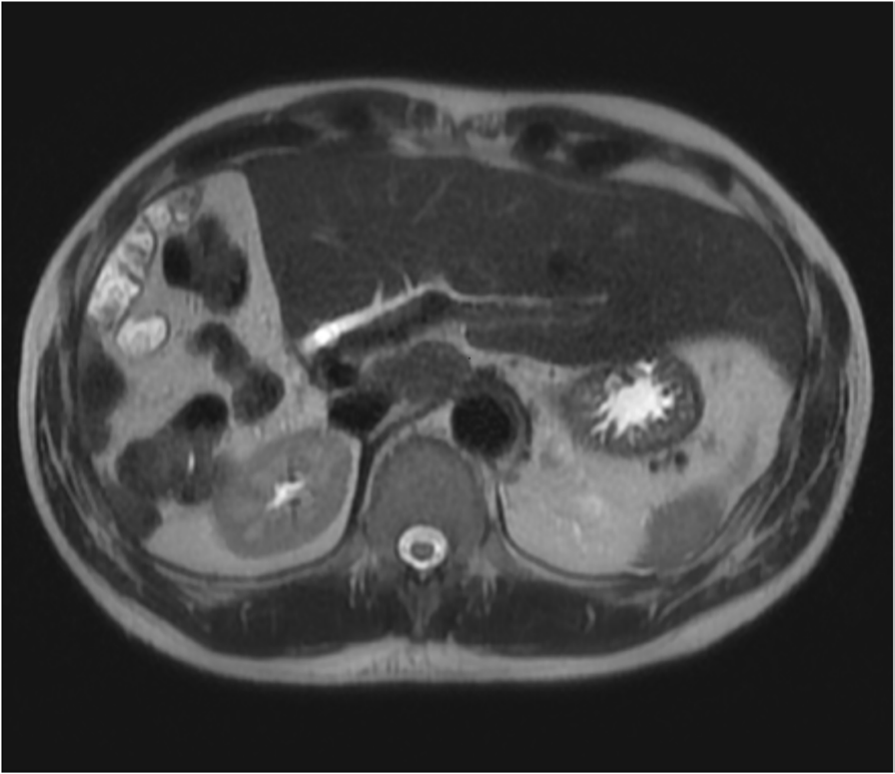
Surveillance MRI of the tumor bed showing no recurrence
